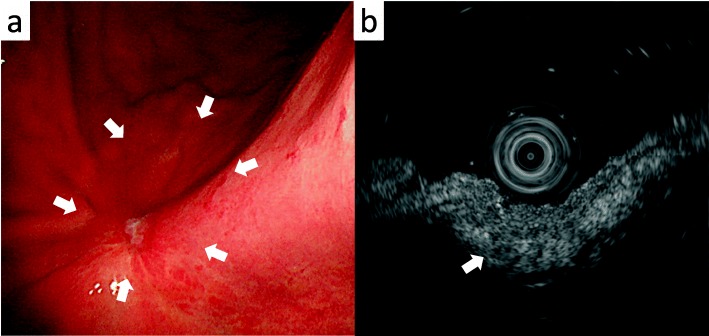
Endoscopic findings. a Endoscopy image showing a type 0–IIc lesion with an ulcer scar on the greater curvature side of the fundus (arrow). b Endoscopic ultrasound showing a poorly demarcated third layer at the ulcer scar (arrow)
